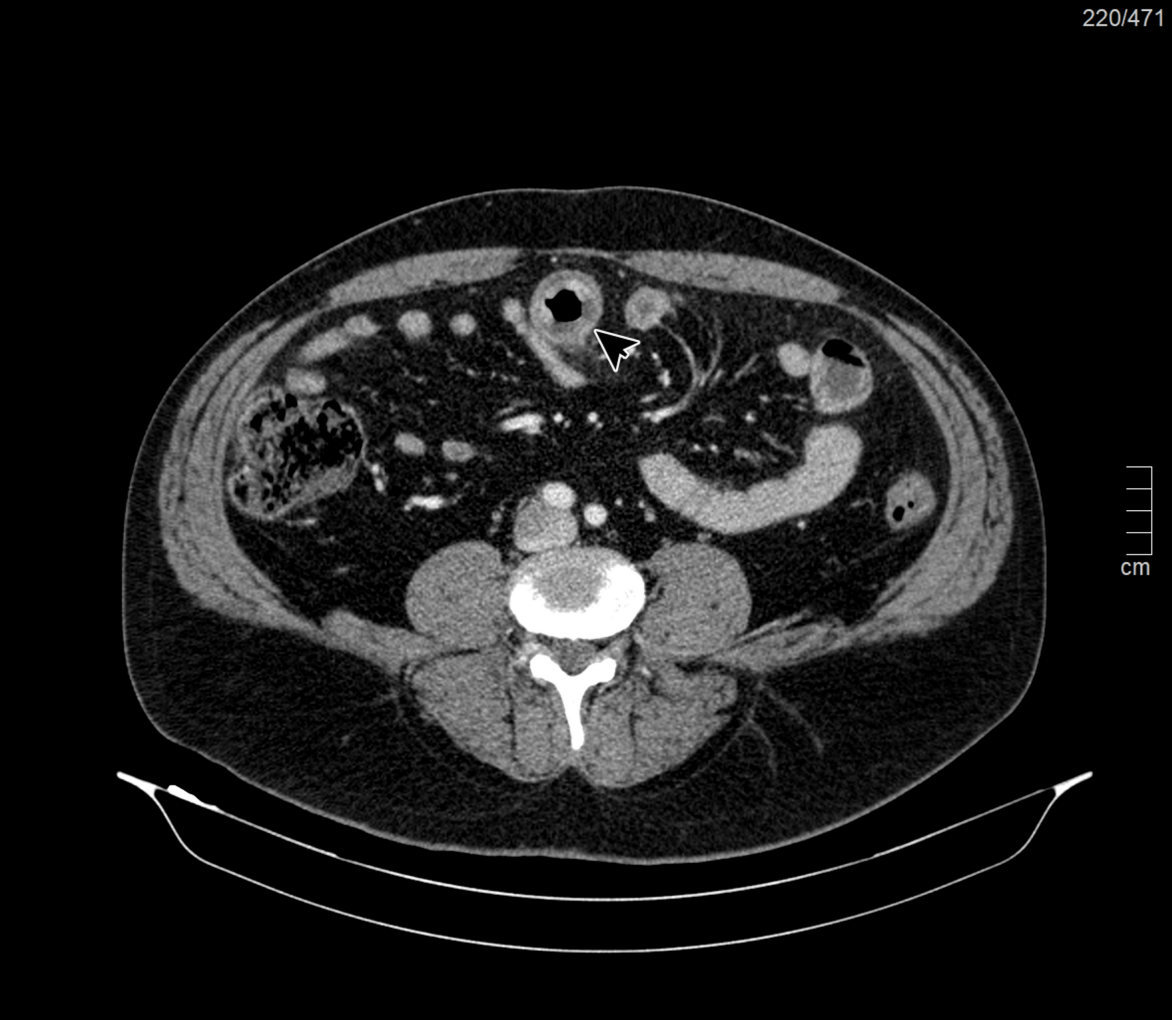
Axial plane of abdominal CT Marked circumferential thickening of the jejunal mucosa is noted with densification of the adjacent peritoneal fat and mesenteric vascular engorgement (arrowhead).
